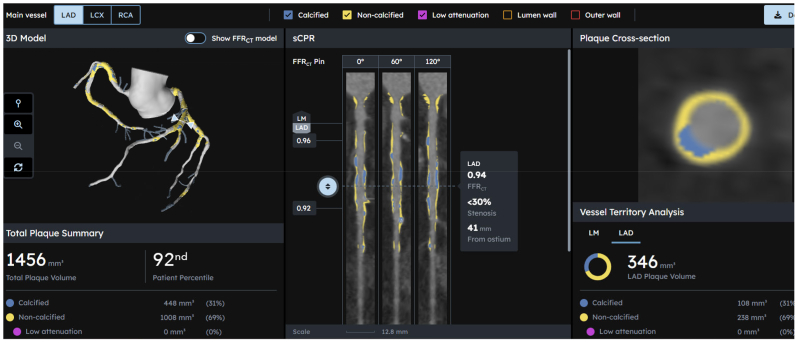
Coronary computed tomography (CT)-derived quantitative plaque analysis (*CT-QPA*). Case example of asymptomatic patient with very high total plaque volume (1456 mm^3^) comprised of noncalcified plaque (1008 mm^3^) and calcified plaque (448 mm^3^) and no evidence of coronary ischemia [CT-derived fractional flow reserve (FFR_CT_) > 0.80 throughout]. Dynamic controls enable interrogating the three-dimensional model and scrolling through each vessel with cross-sections and FFR_CT_ values at any selected site.
